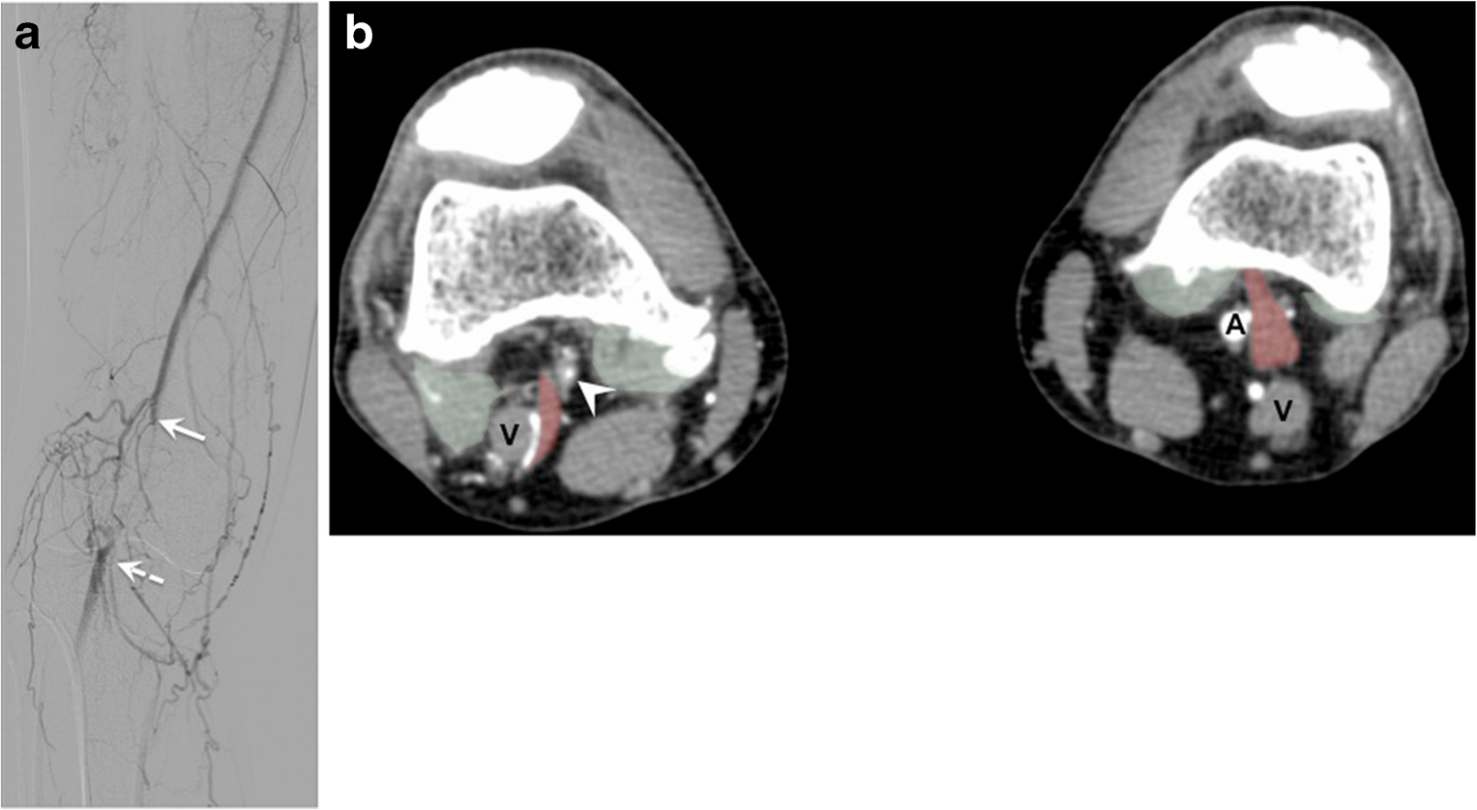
Popliteal artery entrapment syndrome (PAES) in a 52-year-old man with a history of right calf claudication for 2 years. (a) Digital subtraction angiography shows abrupt occlusion of the proximal popliteal artery (*solid arrow*), which reconstitutes at its midportion (*dashed arrow*). A cine sequence is presented in Movie 1. PAES was suspected and patient underwent CT angiography. (b) Axial images of CT angiography reveal severe narrowing of the right popliteal artery (*arrowhead*), with an abnormal muscle slip (*red transparent overlap*) originating from the medial head of the gastrocnemius muscle and coursing between the popliteal vessels (V = popliteal vein). The contralateral side shows a similar appearance of abnormal muscle slip (*red transparent overlap*); however, the left popliteal artery is patent (A). *Bilateral green transparent areas* correspond to normal insertions of the gastrocnemius insertions
